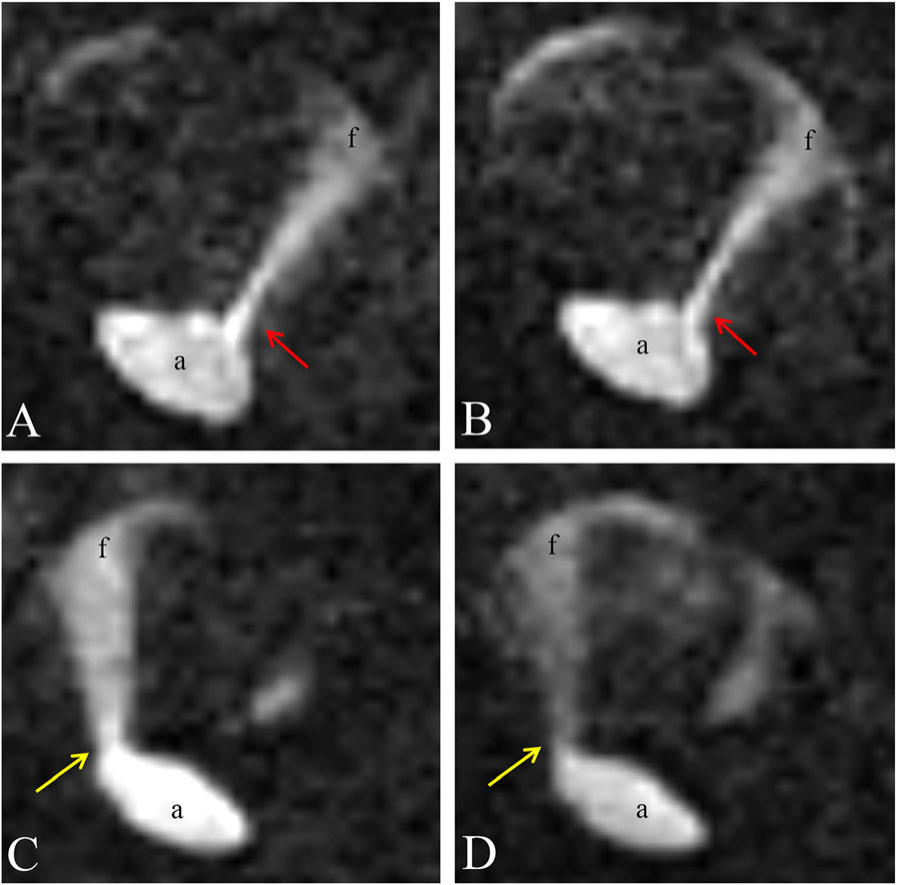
4D (three-dimensional with time dynamic) phase-contrast MRI axial view provides the information of precise location and dynamic blood flow change into the false lumens. In the follow up 4D-PC MRI, a, b revealed the type Ib endoleaks (red arrow) and c, d showed another new arising type III endoleaks (yellow arrow). (a: aorta / f: false lumens)
